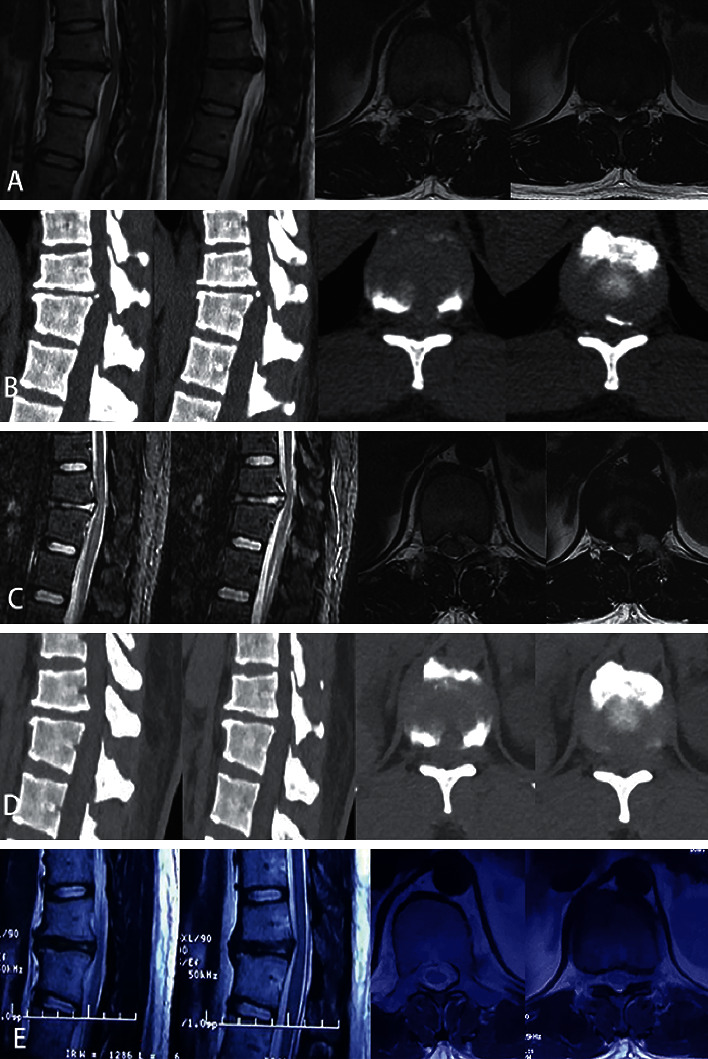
Preoperative MRI (a) and CT (b) scan showing spinal cord compression by calcified disc herniation and posterior vertebral osteophyte at T11-T12 (case 3). Postoperative MRI (c) and CT scan (d) showing sufficient decompression of the spinal cord and the calcified disc was cut off clearly. MRI at the 3-month follow-up (e) showing the spinal cord surrounded by cerebrospinal fluid.
